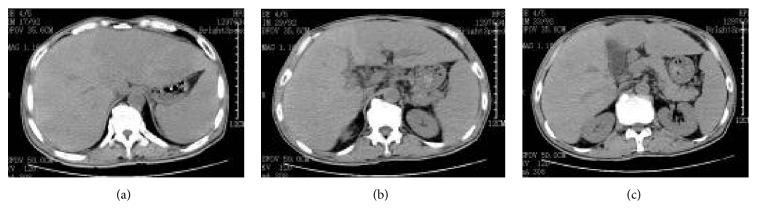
Epigastrium CT before treatment. Note: the liver outline was significantly more prominent. The liver edge was less smooth. Liver parenchymal density was uneven. Patchy low-density shadows were observed, especially in the left live. Multiple quasicircular low-density foci appeared in the right lobe of the liver. The spleen was enlarged to about seven rib units. The density was uniform.
